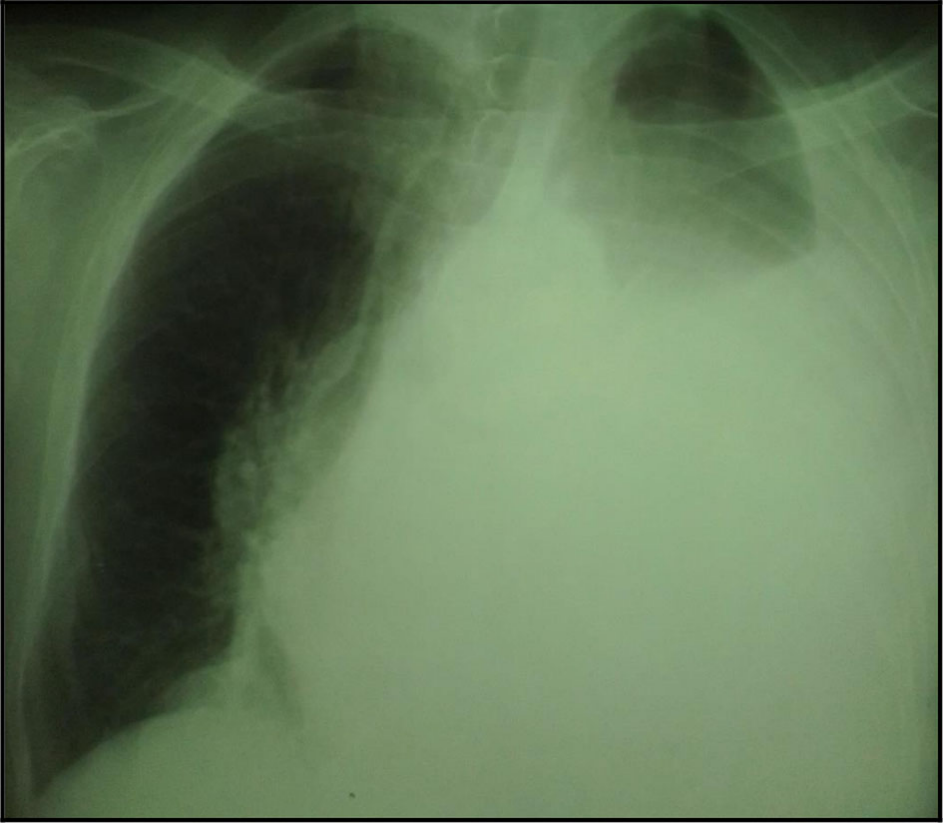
Chest X-ray showing a pleural opacity in the left chest repressing the trachea and the mediastinum.
